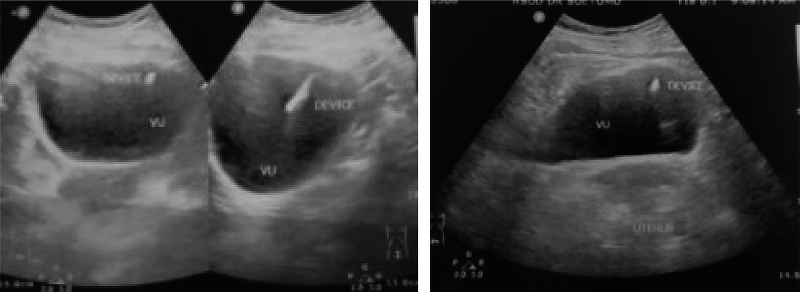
Ultrasound view of the migrated IUCD in the bladder.
